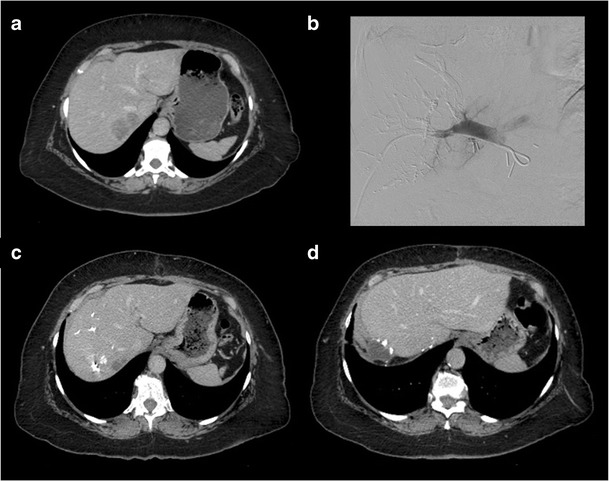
Portal vein embolisation prior to right hepatectomy. a Axial enhanced CT image shows colorectal liver metastasis involving segments V, VI, VII (only VII shown). b Final portogram of embolised portal vein branches in segments V through VIII using a Lipiodol-glue mixture. c Axial enhanced CT image obtained 1 month after right PVE showing hypertrophy of future liver remnant. d Axial enhanced CT image of the same patient after right hepatectomy
